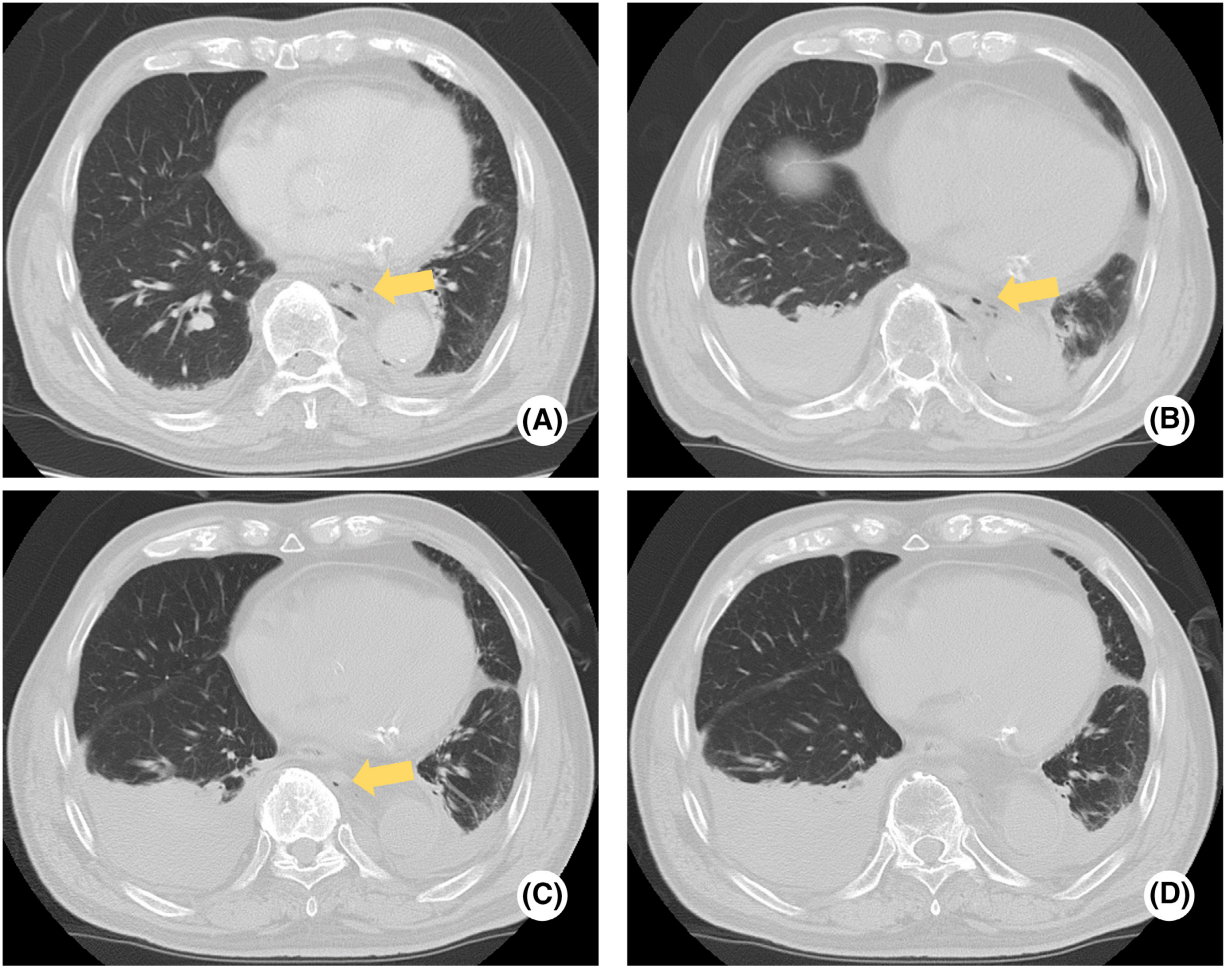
Chest computed tomography (CT) of the patient. Posterior pneumomediastinum (yellow arrows) shown in A, B, and C of the chest CT image. A：Day 1, B：Day 2, C：Day 4, D：Day 9.
